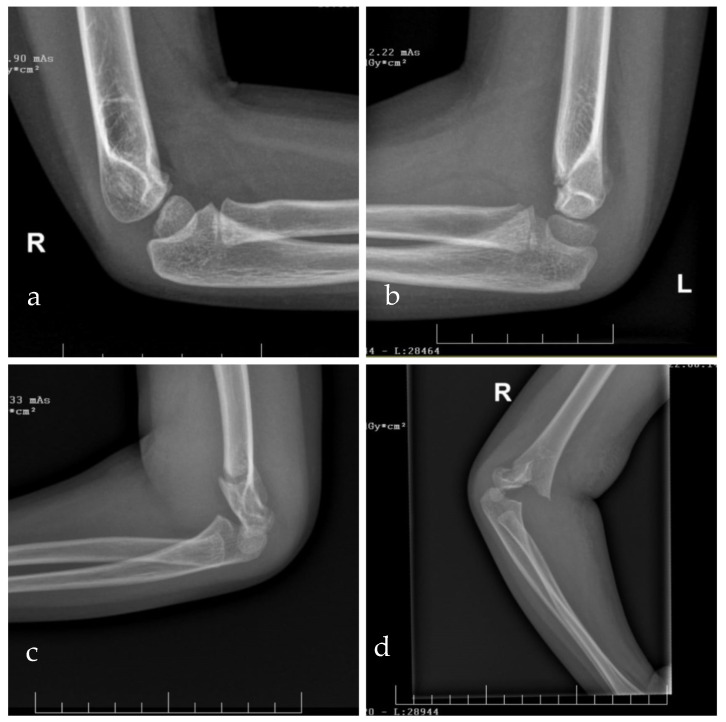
Radiographs of supracondylar humeral fracture according to Gartland classification modified by Wilkins, from our patient cohort. Gartland type I (a), Gartland type II a (b), Gartland type II B (c), and Gartland type III (d).
